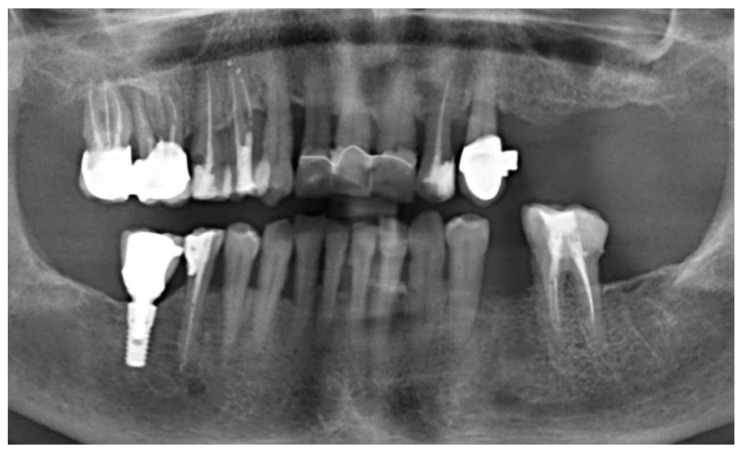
Orthopantomogram X-ray examination of the patient.
